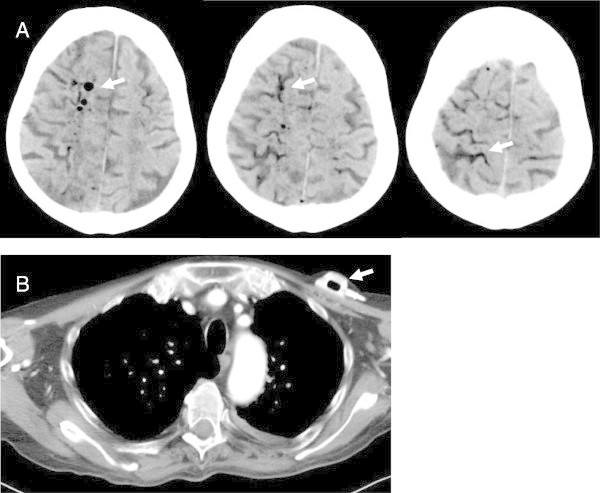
CT in cerebral air embolism via port catheter. Panel A, native axial CT-scan of the brain shows inclusion of air (arrow) within the subarachnoidal spaces more prominent in the right hemisphere. Panel B, CT-thorax shows air in the port catheter (arrow) which is used for parenteral nutrition under home care conditions.
